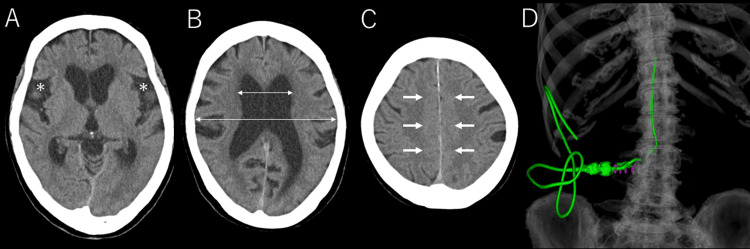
Characteristic head CT findings of idiopathic normal pressure hydrocephalus and LP shunt placement CT: computed tomography; LP: lumboperitoneal Head CT demonstrates typical findings of disproportionately enlarged subarachnoid space hydrocephalus (DESH) associated with idiopathic normal pressure hydrocephalus, including widening of the Sylvian fissures (A, asterisk), ventricular enlargement with an Evans index of 0.34 (B, double arrows), and narrowing of the high convexity and midline sulci (C, arrows). A 3D CT image confirms the placement of the LP shunt (D)
